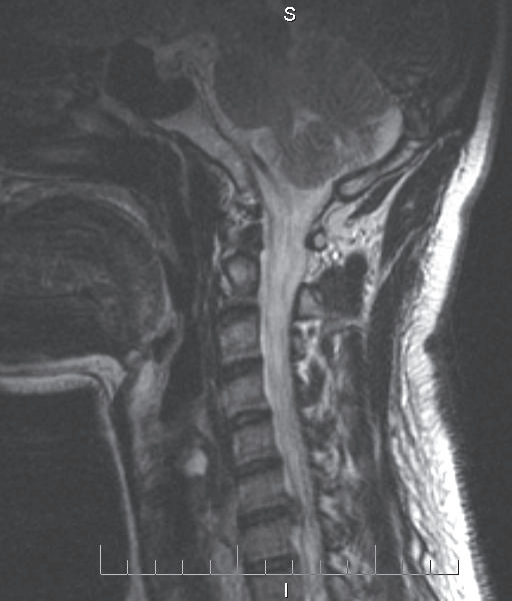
Cervical MRI showing C6-7 right disc herniation
